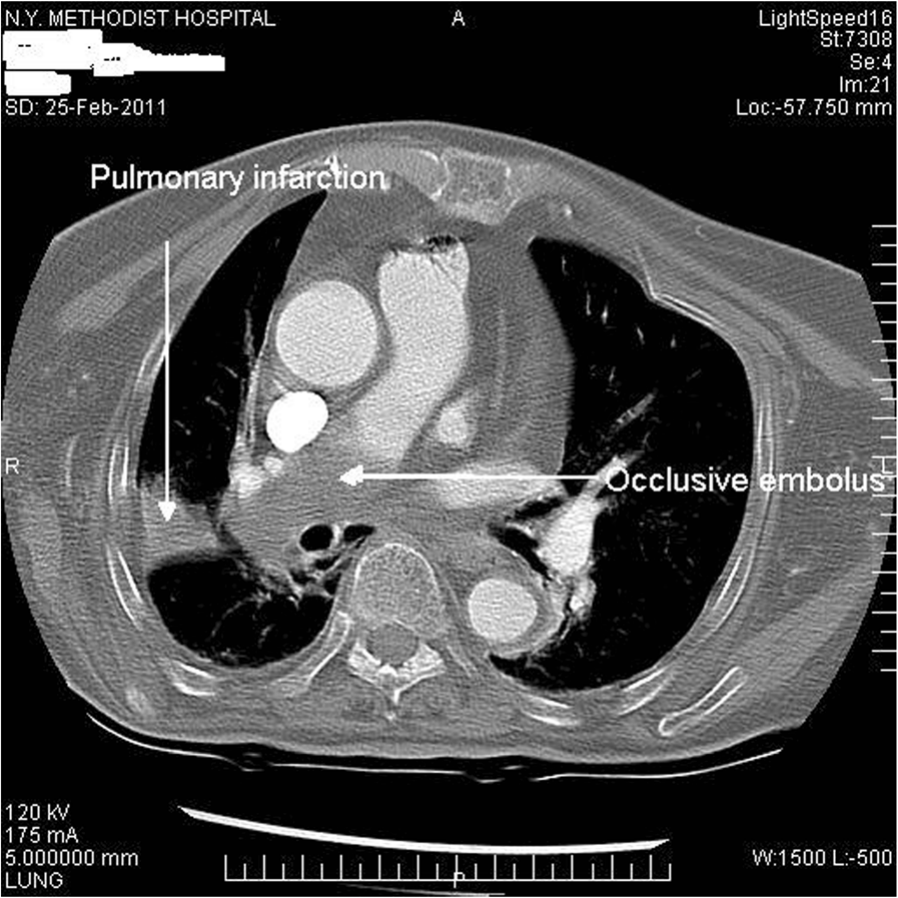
CT scan showing an occlusive pulmonary embolus.
